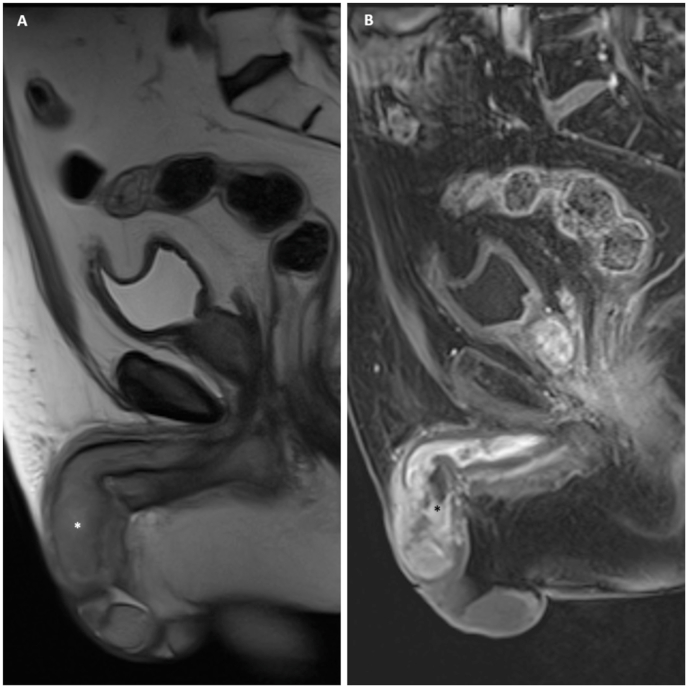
A) Sagittal T2-weighted MR image and B) sagittal post-contrast T1-weighted fat-saturated image showing longitudinal anatomy of penile shaft. Suspected corpora infiltration has been marked as (∗).
